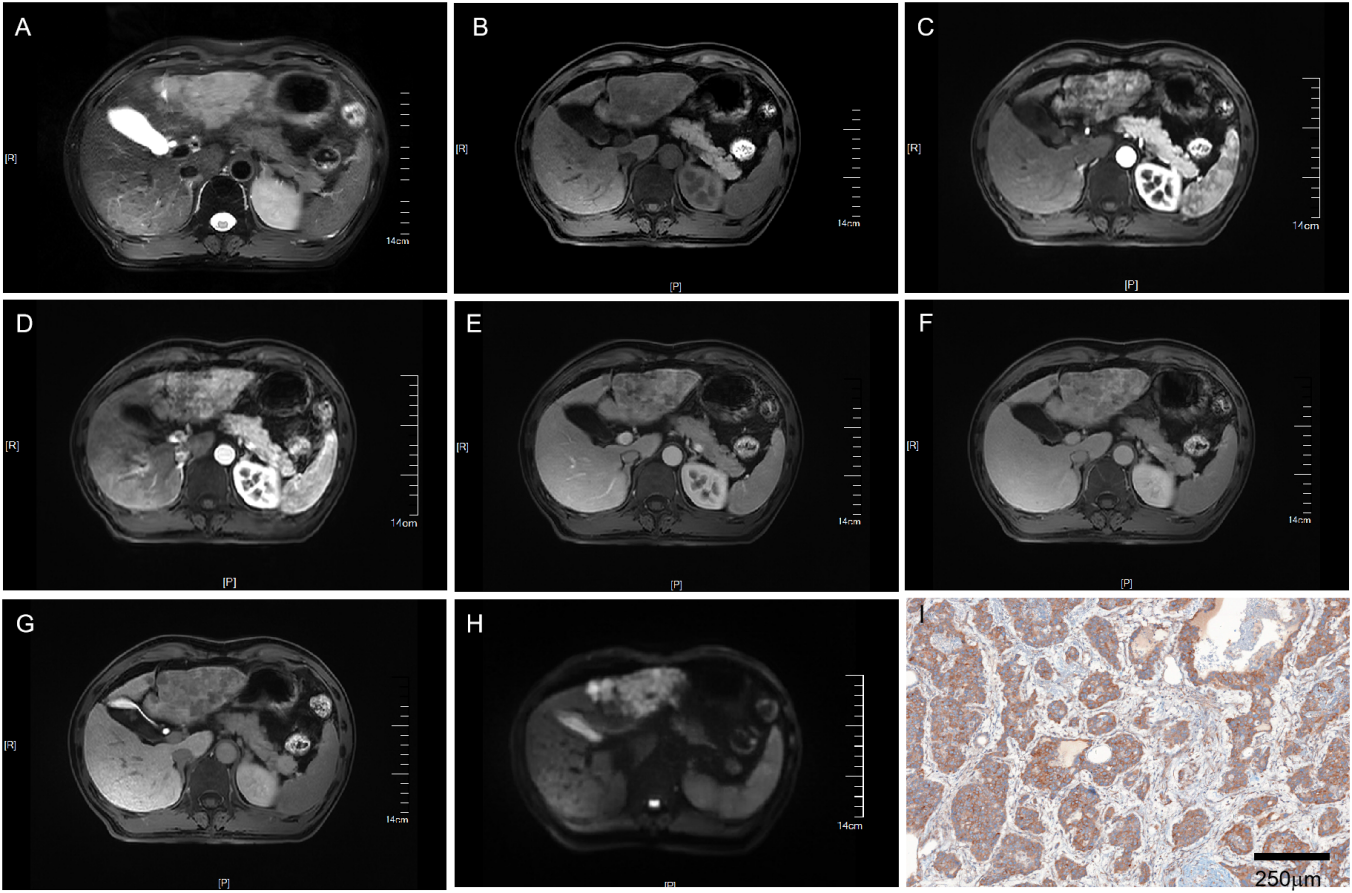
MRI of a VEGFR2-positive HCC patient with LI-RADS 5. Patient summary: a 50-year-old man with HCC in the left lobe of the liver, AFP level of 266ng/ml, lesion dimension on MRI of 8.1cm, and LI-RADS score of 5. (A) Axial T2-weighted MRI, (B) Axial T1-weighted MRI. (C, D) Early (15s) and late arterial phase (25s). (E) Portal venous phase (1min). (F) Delay phase (3min). (G) Hepatobiliary phase (15min). (H) Axial diffusion-weighted MRI. (I) VEGFR2-positivity in pathology. MRI, magnetic resonance imaging; LI-RADS, liver imaging reporting and data system; AFP, alpha-fetoprotein; VEGFR2, vascular endothelial growth factor receptor 2.
